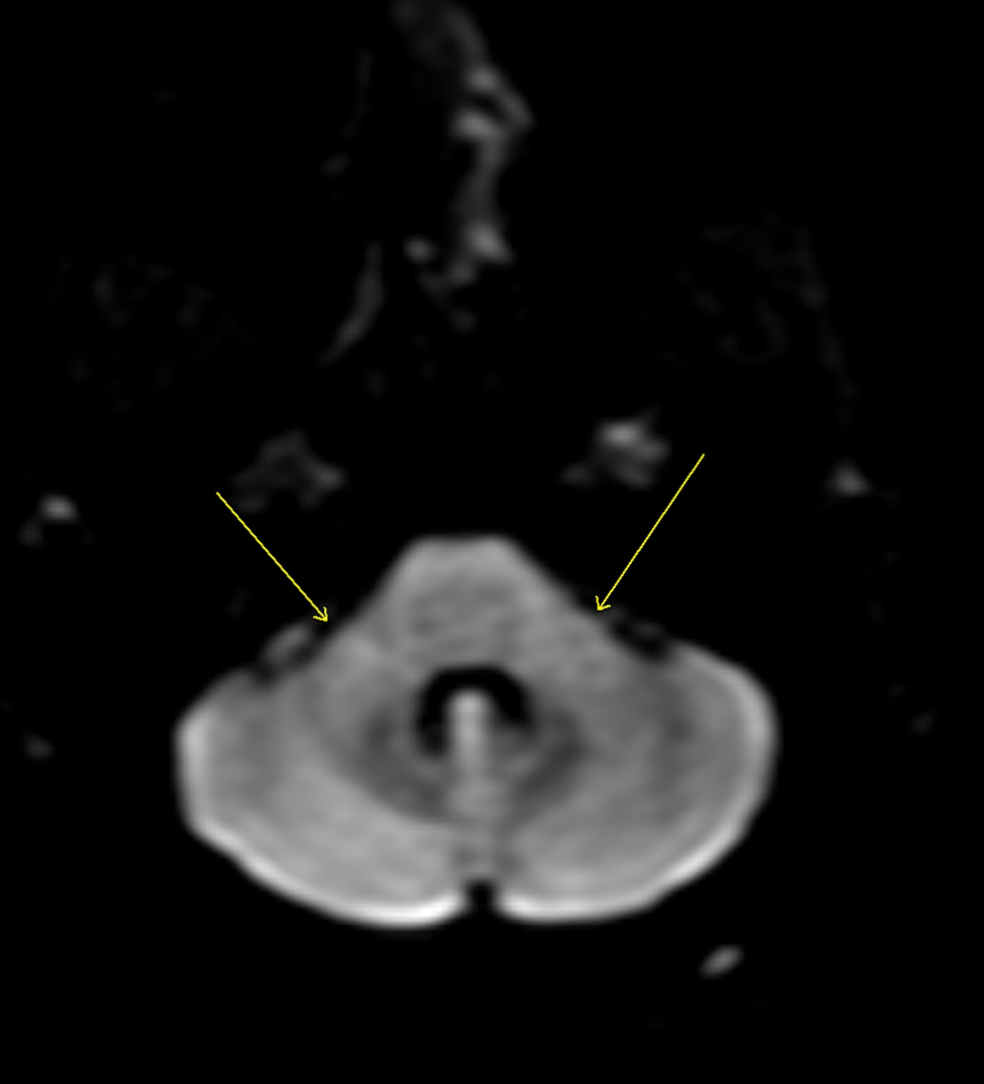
Post-contrast diffusion-weighted MRI when the patient presented. The arrows show brachium pontis.
